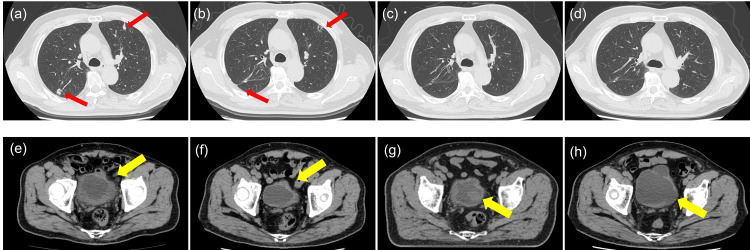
Representative case managed with avelumab and radiation (Case 2) Computed tomography (CT) scan revealed shrinkage and increase of the primary bladder cancer (yellow arrow) and lung metastasis (red arrow) in case 2 (a, e). Images at pretreatment (b, f), after four cycles of GC (c, g), and after 54 cycles of avelumab therapy (d, h). After additional radiation combined with avelumab therapy, there was maintenance of tumor shrinkage for the primary bladder cancer and lung metastasis for 27 months.
